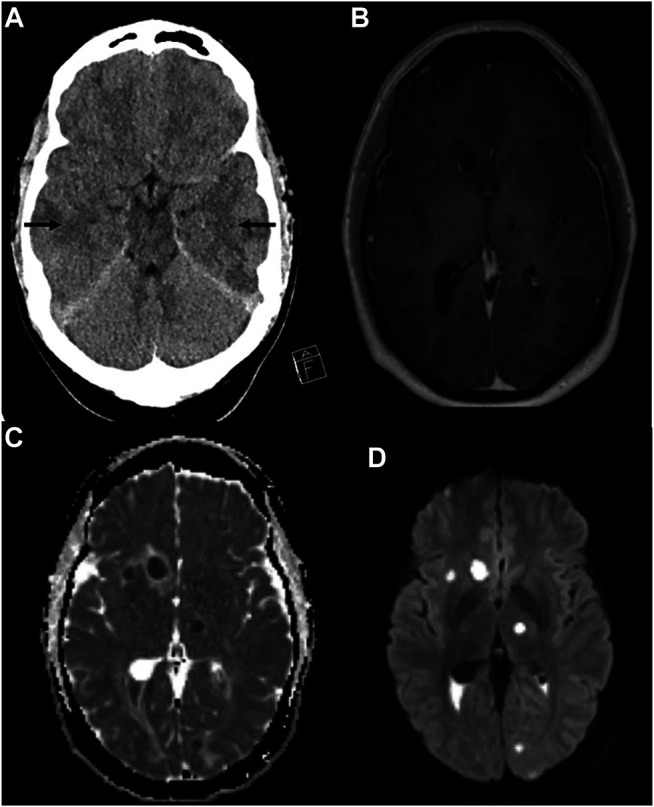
Computer tomography showing hypodense areas bilaterally in the temporal lobes indicating edema (arrows) (A). MRI of the brain with T1WI with gadolinium contrast (B), apparent diffusion coefficient (ADC) image (C), and diffusion-weighted imaging (DWI) (D). Multiple peripherally contrast-enhancing lesions with perilesional edema were found both supra- and infratentorial (B). A thin ependymal contrast enhancement is found. Diffusion imaging (C,D) show reduced signaling in the parenchymal lesions and in the content of the posterior horns, indicating multiple abscesses and ventriculitis.
